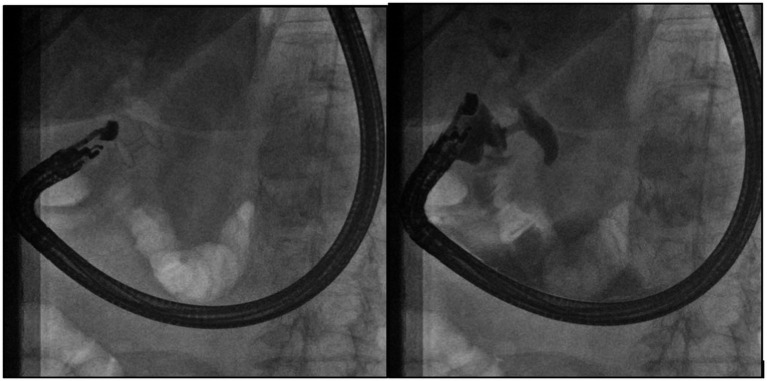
Abdominal computed tomography of EUS-GBD. In the left image, the LAMS was deployed between the duodenum and CBD. In the right image, the contrast flowed thorough the LAMS into the CBD.
